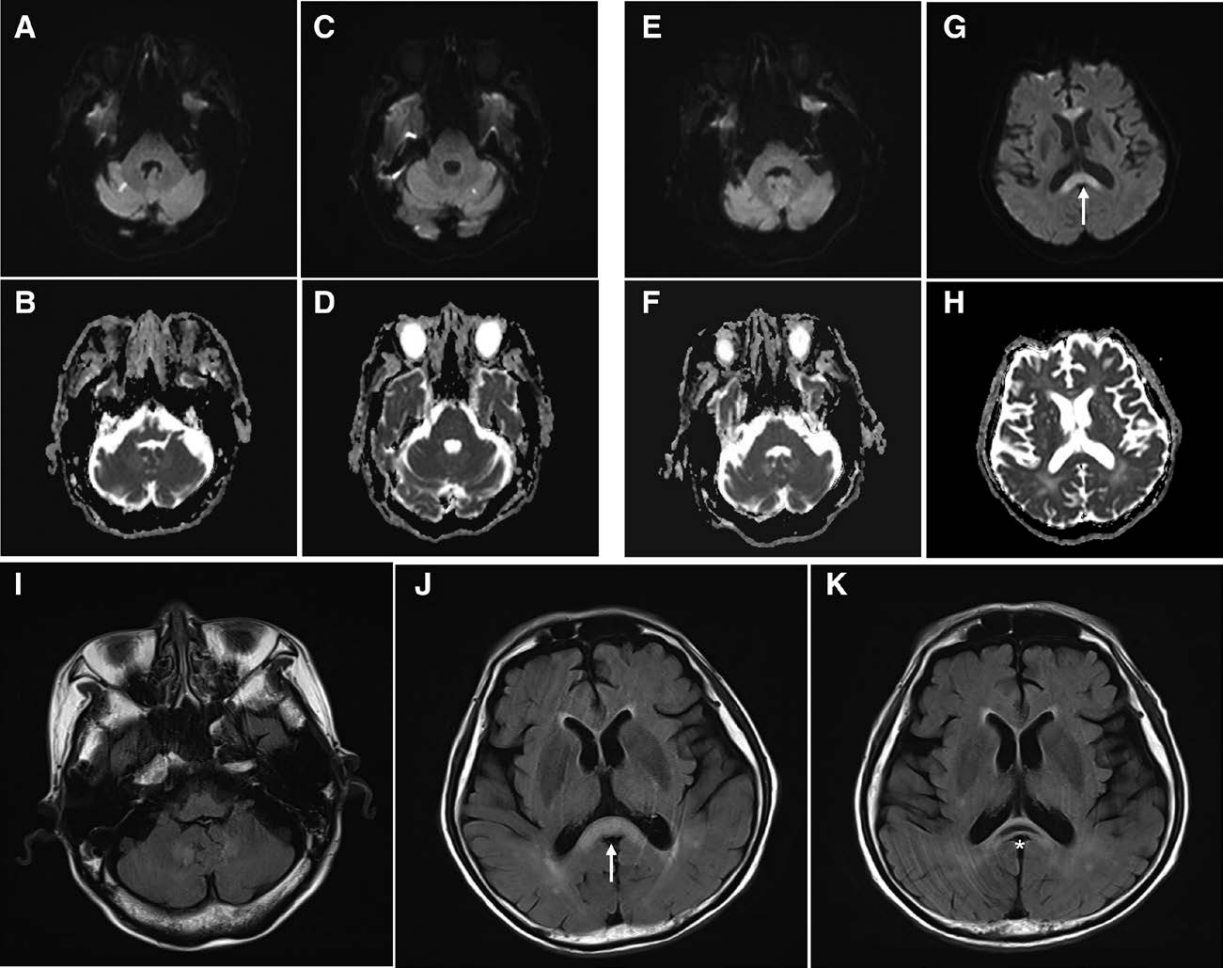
Initially obtained brain MRI of the patient showed an acute ischemic stroke in the bilateral cerebellar hemisphere (A, B, C, D). One month after the initial brain MRI, a follow-up brain MRI was performed, which showed diffusion restriction in DWI images in the corpus callosum (white arrow), but no abnormal signals in both dentate nuclei (E, F, G, H). In T2-weighted FLAIR images, the high signal intensity of the corpus callosum was depicted (white arrow, I, J), and the second follow-up MRI showed encephalomalacia in the corpus callosum (asterisk, K). DWI = diffusion-weighted images, FLAIR = fluid-attenuated inversion recovery, MRI = magnetic resonance imaging.
